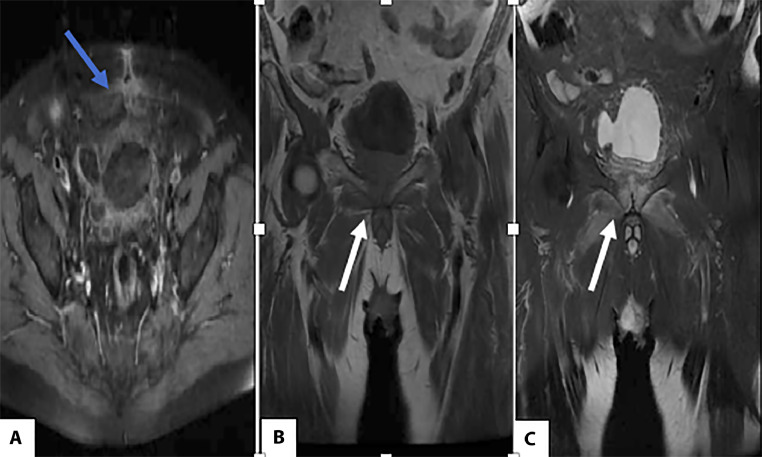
Axial section, post-contrast T1-weighted sequence (A). Infiltration of the pelvic region following urologic surgery. (B) and (C): Signal abnormality of the pubic symphysis, with low signal on T1 and high signal on T2, consistent with bone marrow edema.
